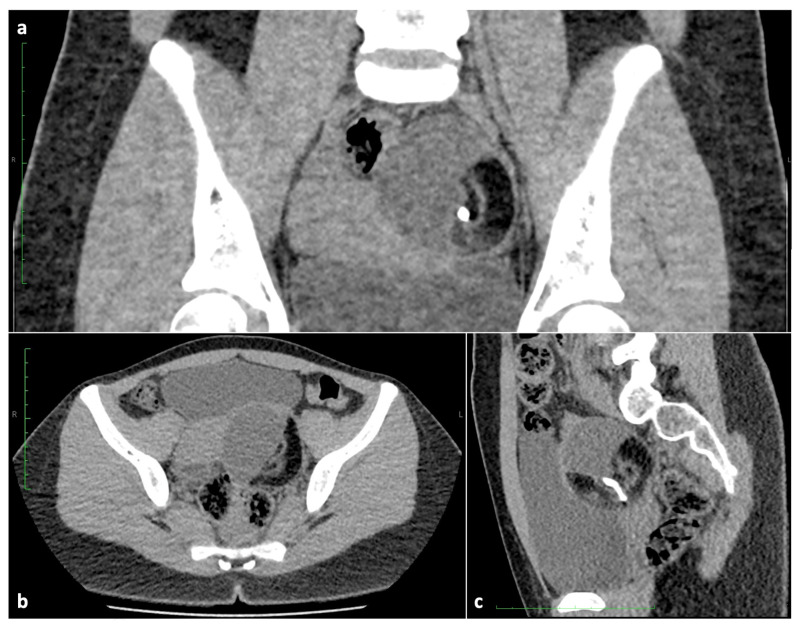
Computed tomography: (a) coronal, (b) axial and (c) sagittal scans of a 17-year-old patient showing a mature teratoma that contains fatty components, a Rokitansky nodule and a calcification.
